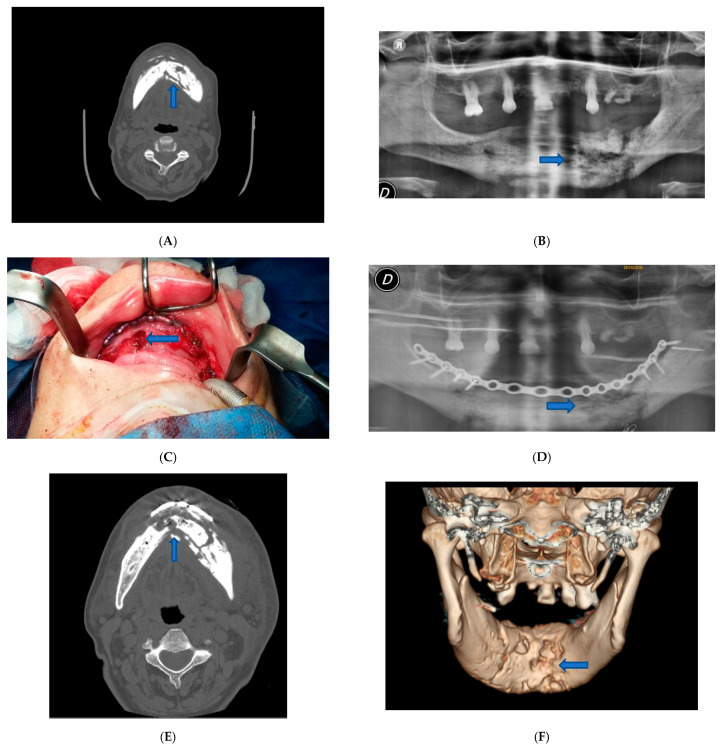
Patient 1. (A) Pre-operative CT scan image showed lithic area in the mandibular horizontal branches, which appeared subverted and fractured on the left side. (B) Pre-operative panoramic X-ray image of the dental branch revealed lithic area. (C) Epi-mucosal fixation performed using SMART Lock screws and plates without elevating the mucoperiosteal flap. (D) Post-operative panoramic X-ray image of the dental branch. (E) Post-operative CT scan image. (F) Post-operative CT scan 3D reconstruction.
